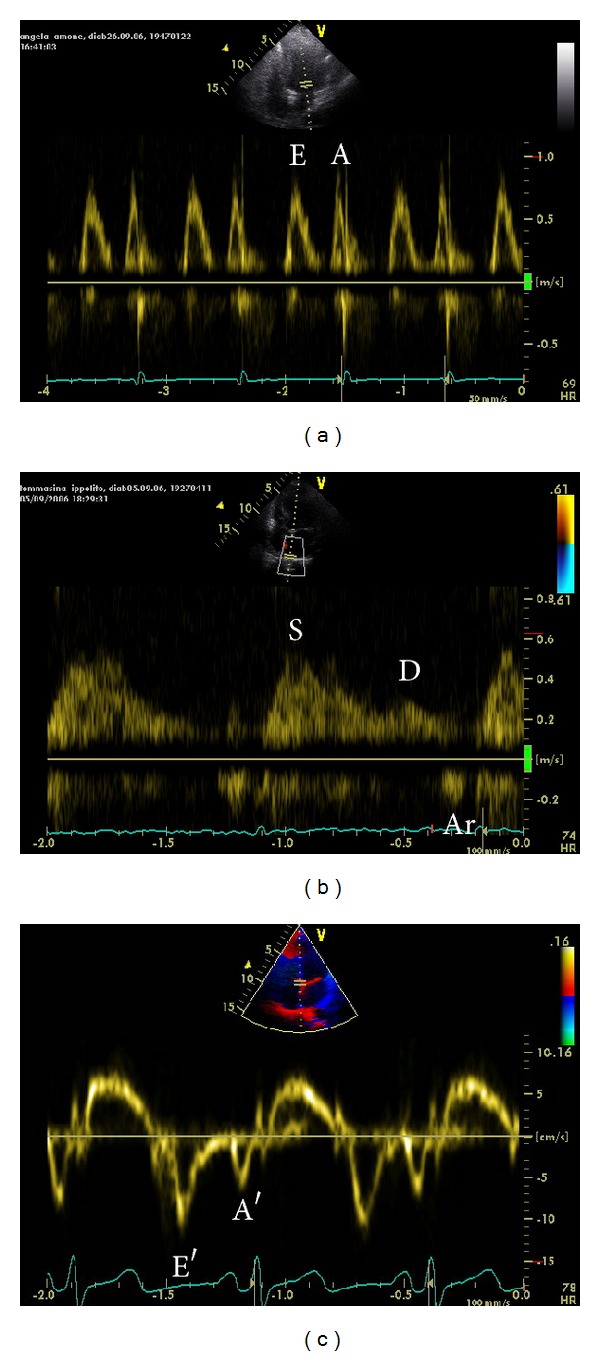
Atrial contraction: wave (a) at mitral inflow (a), peak retrograde diastolic (Ar) velocity at pulmonary venous flow (b) by pulsed Doppler, and late diastolic (A′) velocity by pulsed wave tissue imaging (c).
